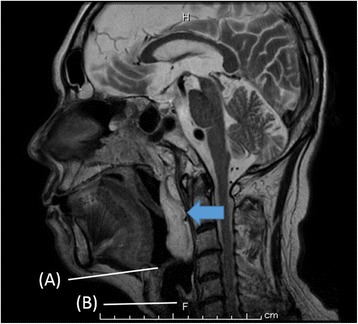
Mass filled within the right nasal cavity to the nasopharynx (MRI scans on head). Arrow: Tumor. a Epiglottis. b Trachea
